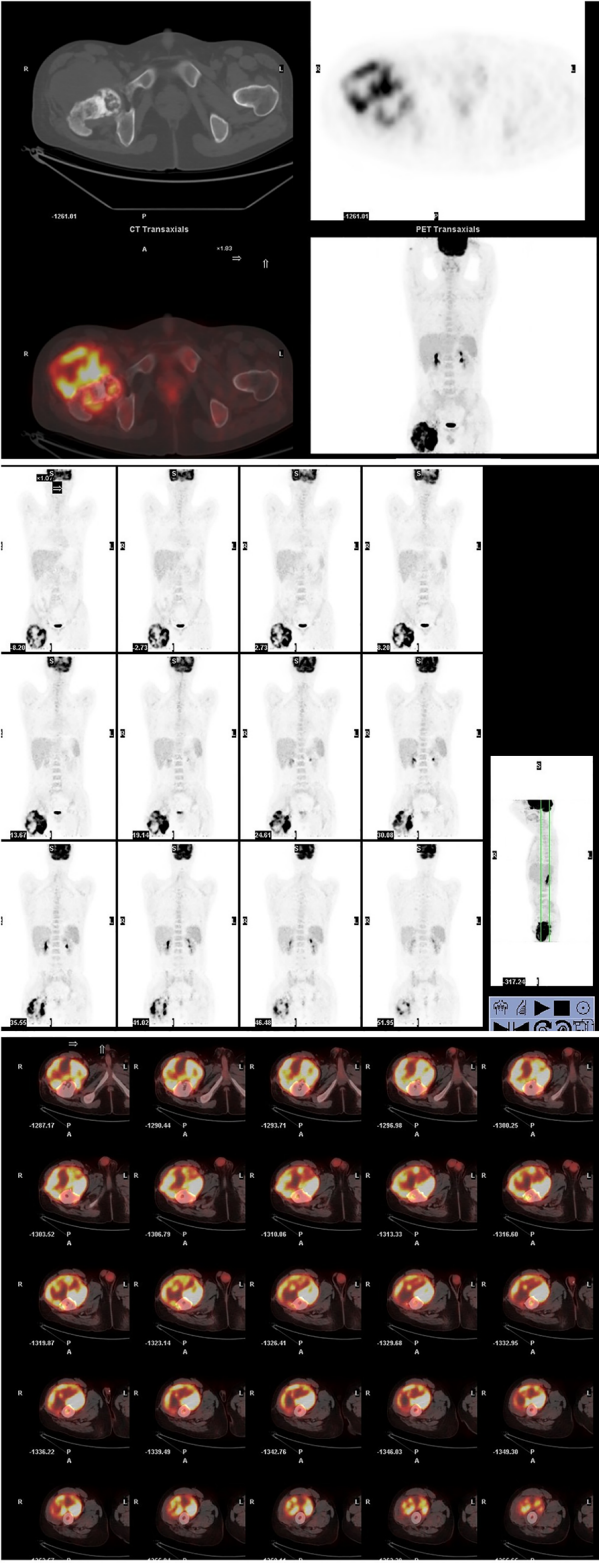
A whole-body tomography positron-emission tomography and computed tomography using ^18^F-fluorodeoxyglucose (^18^F-FDG) demonstrated a huge cauliflower-like appearance skeletal lesion with very high fluorodeoxyglucose uptake (SUV max 8.0) in the upper segment of right femur. It was noted that the ^18^F-FDG avidity was not detected in the lymph nodes or any internal organs.
